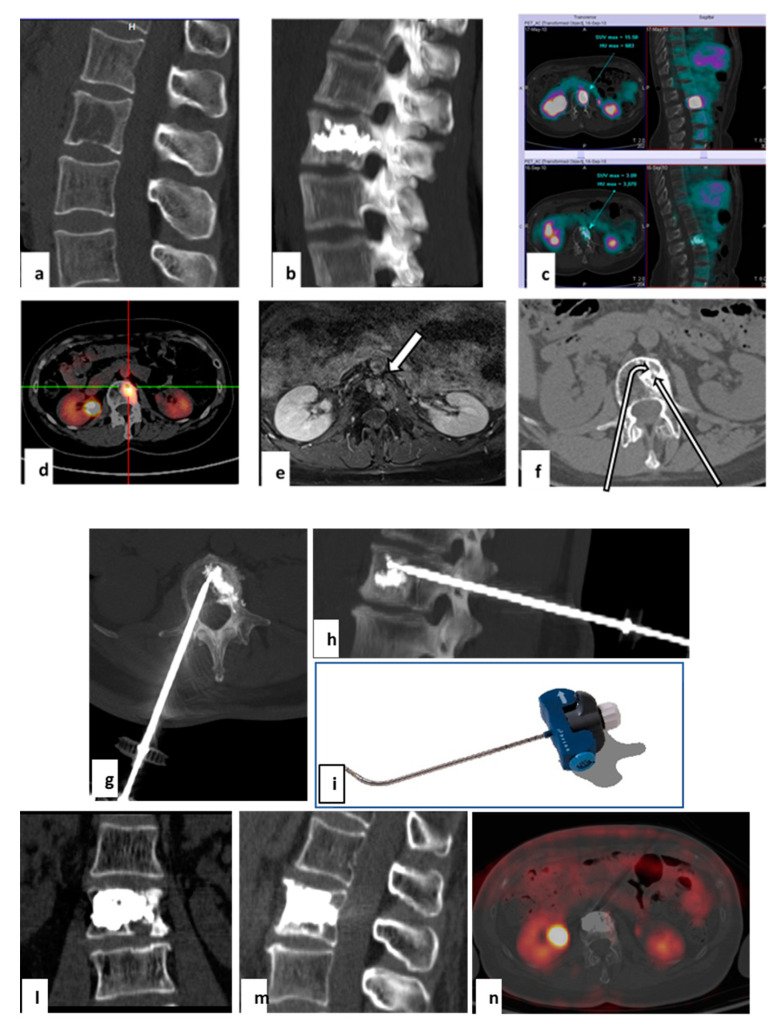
Treatment with tRFA and VA of a recurrent metastasis in a 57-year-old woman with a single L2 lytic metastasis from breast cancer. The metastasis had been treated previously with microwave ablation. Sagittal CT reconstruction showed a large lytic metastasis of L2 treated with microwave ablation (a). Sagittal CT obtained with MIP technique demonstrated the results of vertebral augmentation (b). PET-FDG performed before and 4 months after treatment demonstrates complete absence of metastasis uptake (c). Vertebral tumor recurrence 12 months after the microwave ablation treatment. FDG-PET demonstrated intense uptake of the vertebral body, anterior to the cemented area (d). T1 MRI with fat suppression acquired after gadolinium infusion revealed an area of contrast enhancement related to recurrence (e). Cement interposition hindered access to metastasis by left transpeduncular route with straight needle (straight arrow). The lesion can be reached by contralateral transpeduncular approach with a steerable needle (tSTAR) (f). Axial and coronal CT scans reconstructed with MIP technique (g,h) after tRFA navigation system placement (i). Coronal and sagittal CT scans after treatment of the second vertebroplasty (l,m). PET-FDG performed after 6 months demonstrates absence of metastasis uptake (n).
